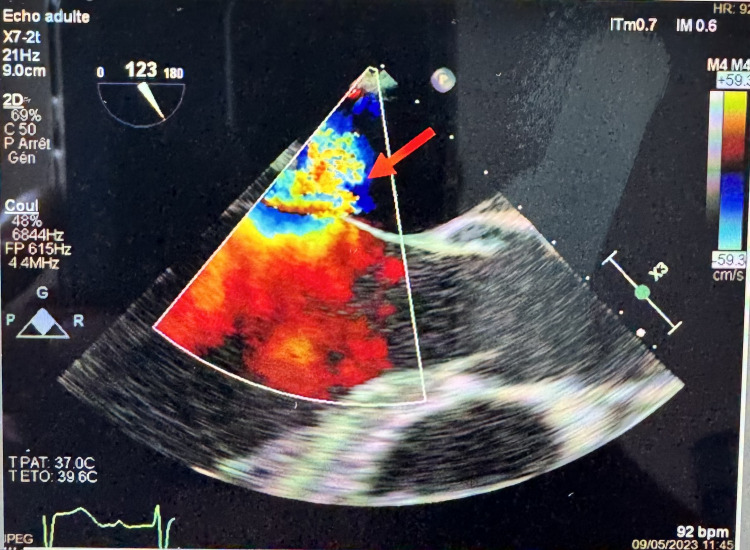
Transoesophageal echocardiography 3 chamber view demonstrating the severity of the mitral regurgitation
